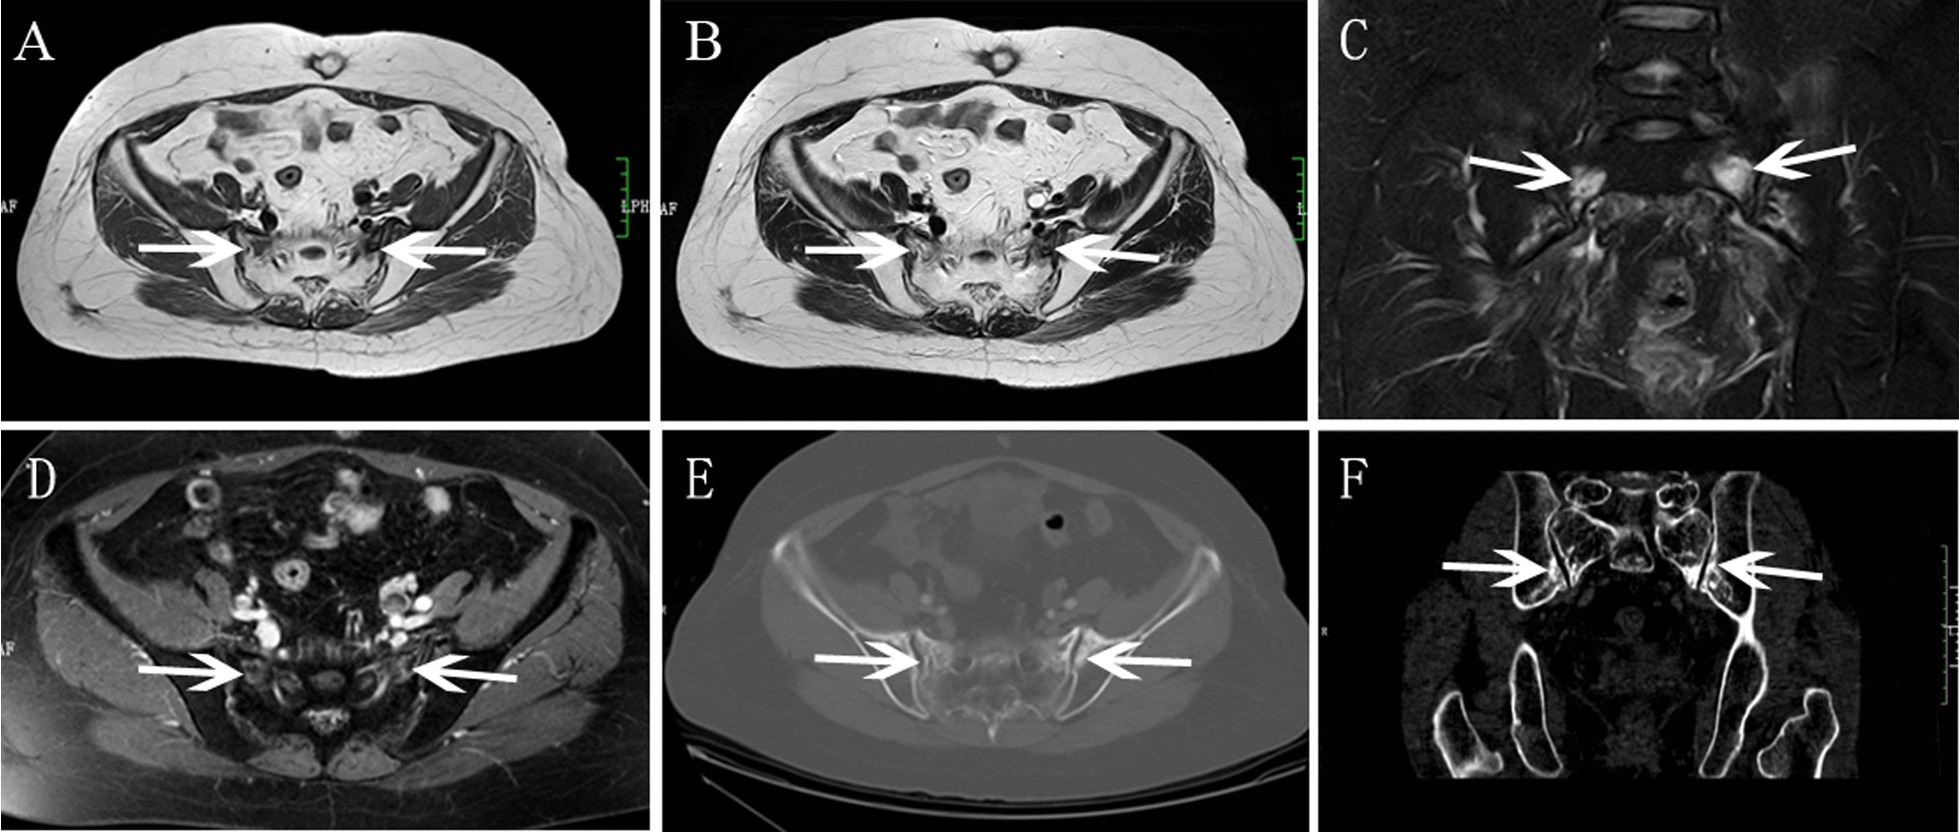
Bilateral SIFs had coexistent bilateral ilium fractures in a 70-year-old woman with cervical cancer after radiotherapy. A, B Axial T1WI and T2WI showed bilateral sacrum low signal (arrow). C Coronal FS-T2WI showed bilateral sacrum and ilium hyperintensity, bilateral sacrum with severe bone marrow edema. D Axial enhanced T1WI showed mild contrast enhancement in bilateral sacrum. E, F. Axial and coronal CT image showed osteosclerosis change
